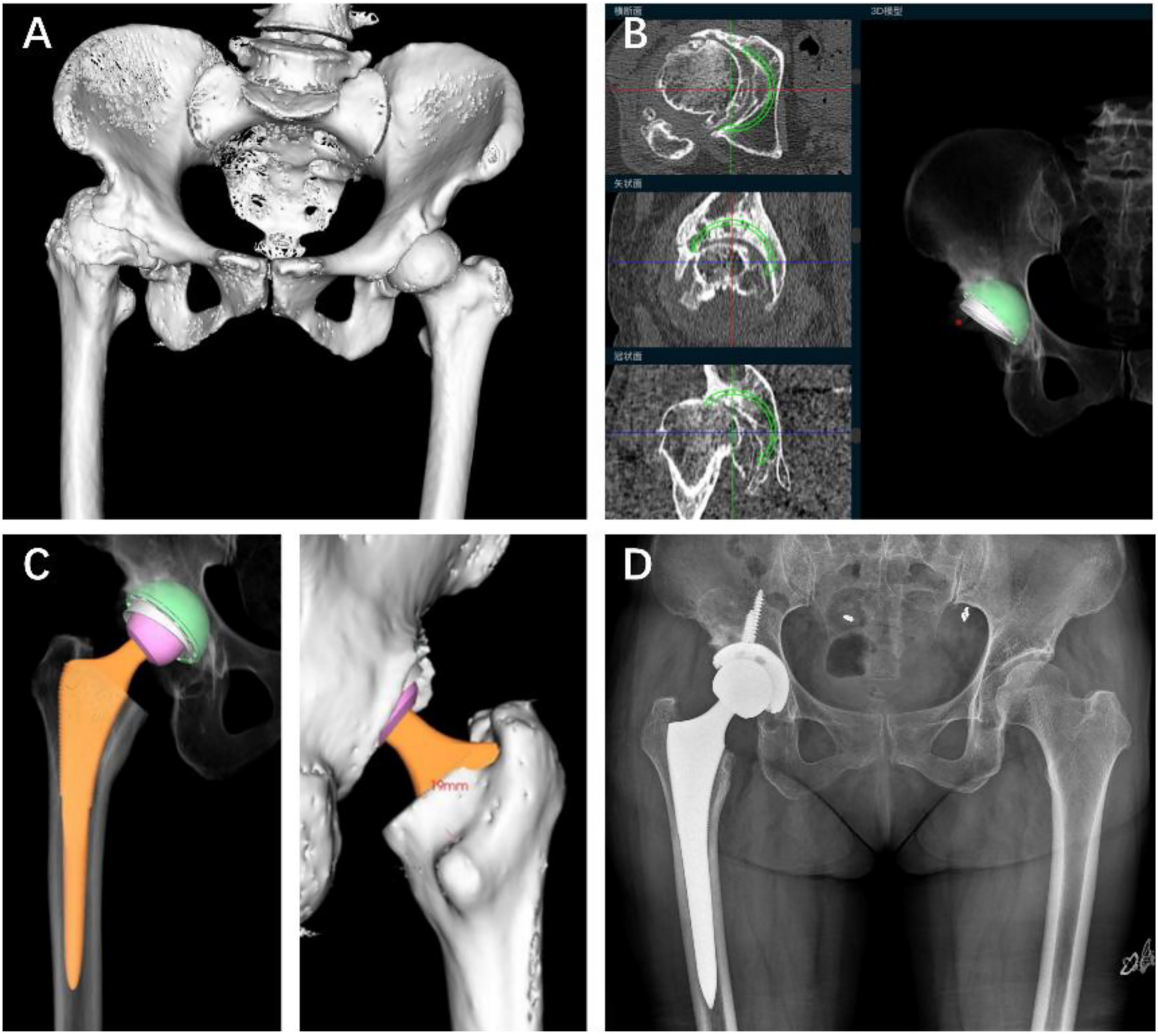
AI-assisted 3D preoperative planning in a Crowe II DDH-HOA case. (A) 3D CT reconstruction. (B) Planned acetabular cup (Pinnacle, size 50). (C) Planned femoral stem (Summit, size 6). (D) Postoperative radiograph showing the final position of the planned implants.
